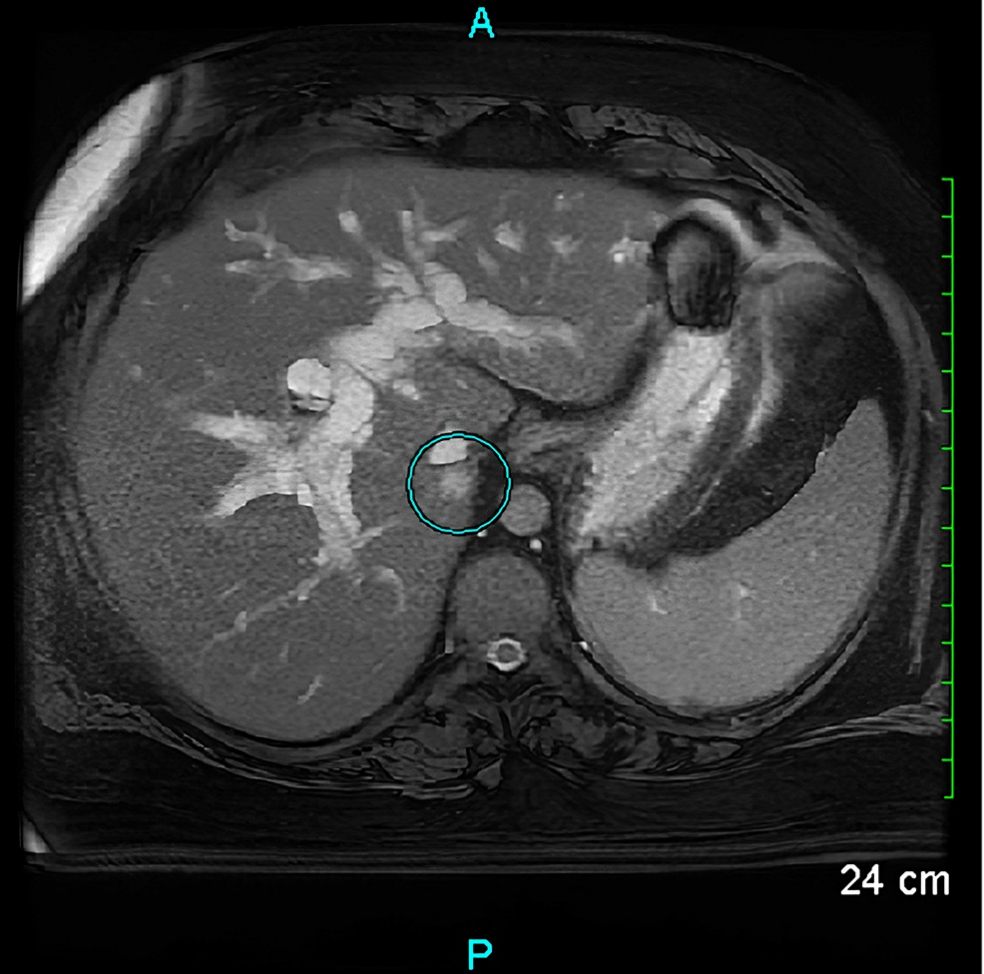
Magnetic resonance imaging of the abdomen with contrast showing heterogenous irregular mass lesion at the periampullary region measuring approximately 2.5 cm
